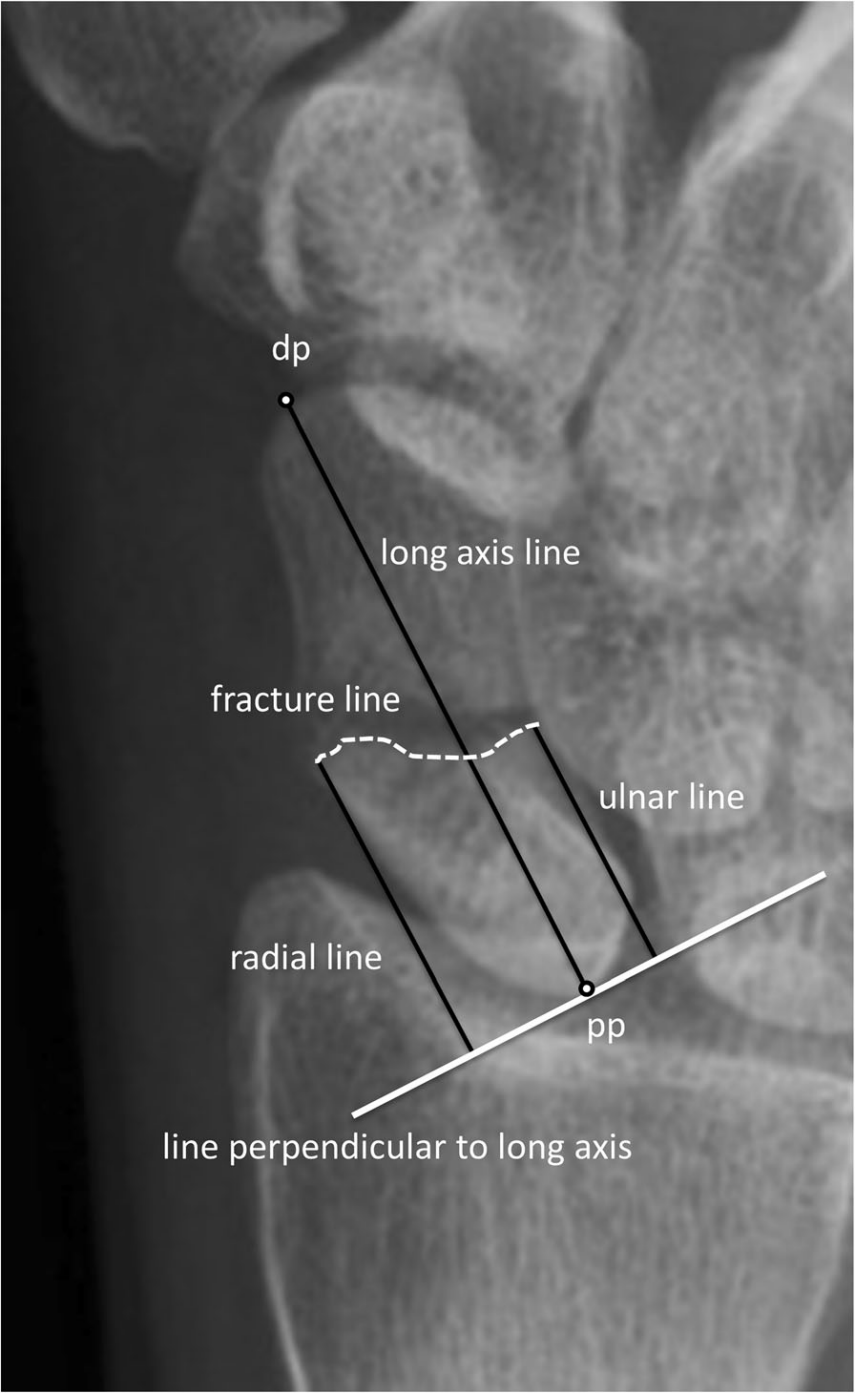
Plain radiograph with the key points relating to ‘long axis’ measurement marked. Detailed legend - a scaphoid fracture with the distal point (dp), proximal point (pp), the long axis line, the fracture line, a line perpendicular to the long axis, and the radial and ulnar lines
